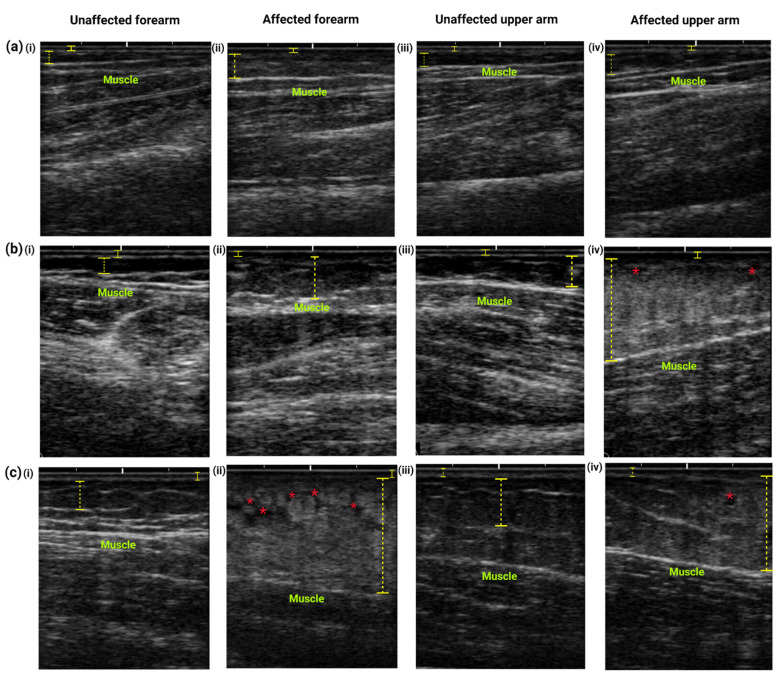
Typical images of ultrasound examination of the (i) unaffected forearm, (ii) affected forearms, (iii) unaffected upper arm and (iv) affected upper arm performed on women with (a) mild and (b,c) moderate to severe stage lymphedema. The structures of the skin measured included the dermis and epidermis layers (yellow straight lines) and the subcutaneous layer (yellow discontinuous lines). The images of the unaffected forearm and upper arm display typical skin structure with normal anatomical structures of the dermis, subcutaneous, and muscle layers. Mild lymphedema is characterized by thickening of subcutaneous layer at the (a(ii)) forearm and (a(iv)) upper arm. In contrast, tissue changes occur mainly at the subcutaneous area, as lymphedema progresses. As demonstrated in (b,c), tissue thickness increased in the affected arms (b(ii,iv) and c(ii,iv)) due to accumulation of fluid in the subcutaneous tissue (indicated by hyperechogenic tissue), which is associated with higher stages of lymphedema. A blurring interface between the subcutaneous and dermis layers is also observed in the affected arms (b(ii,iv) and c(ii,iv)). Prominent subcutaneous edema and fat lobules give the cobblestone or lattice pattern (red asterisks), indicating soft tissue changes that often occur in the subcutaneous layer.
